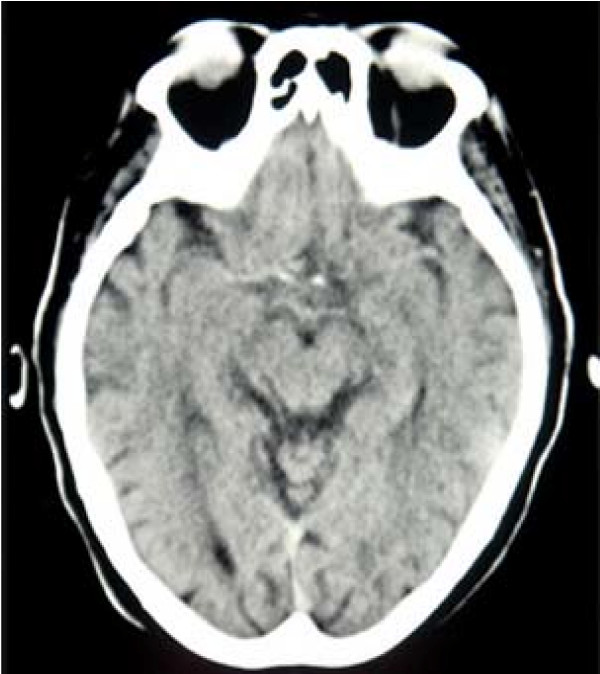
Normal non-injected computed tomography brain scan.
